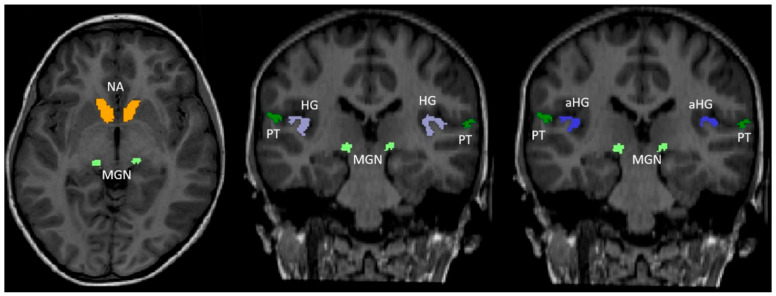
Anatomical structures used in the FreeSurfer analysis overlaid on a coronal reformat of the T1-weighted sequence. Axial and two coronal reformat of the T1-weighted sequence show the color-coded hearing-related brain structures we studied: Heschl’s gyrus (HG, purple, anterior HG (aHG, blue), planum temporale (PT, dark green), medial geniculate nucleus (MGN, light green), and nucleus accumbens (NA, orange).
